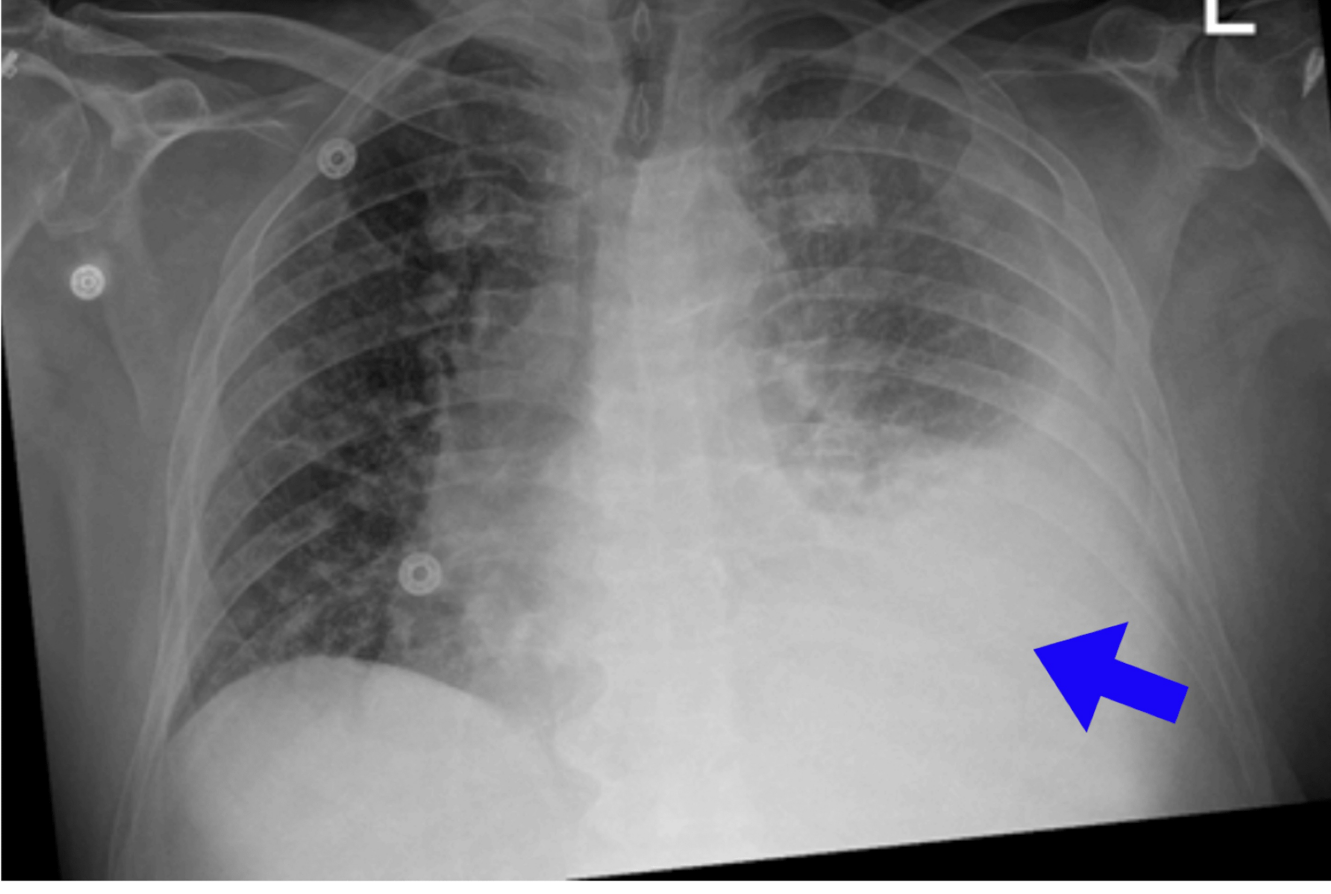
Chest X-ray showing a left-sided pleural effusion
